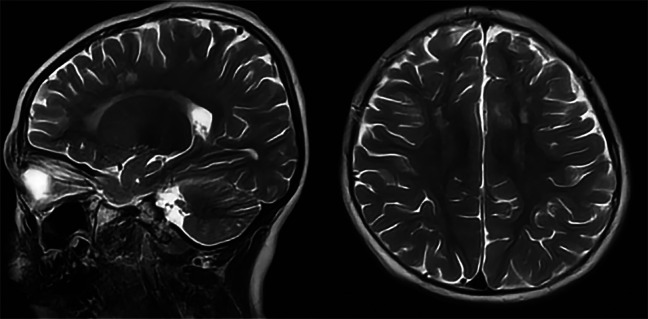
Axial and sagittal T2-weighted MRI of patient 1 showed diffuse high signal intensity within the white matter of the bilateral cerebral hemispheres.
